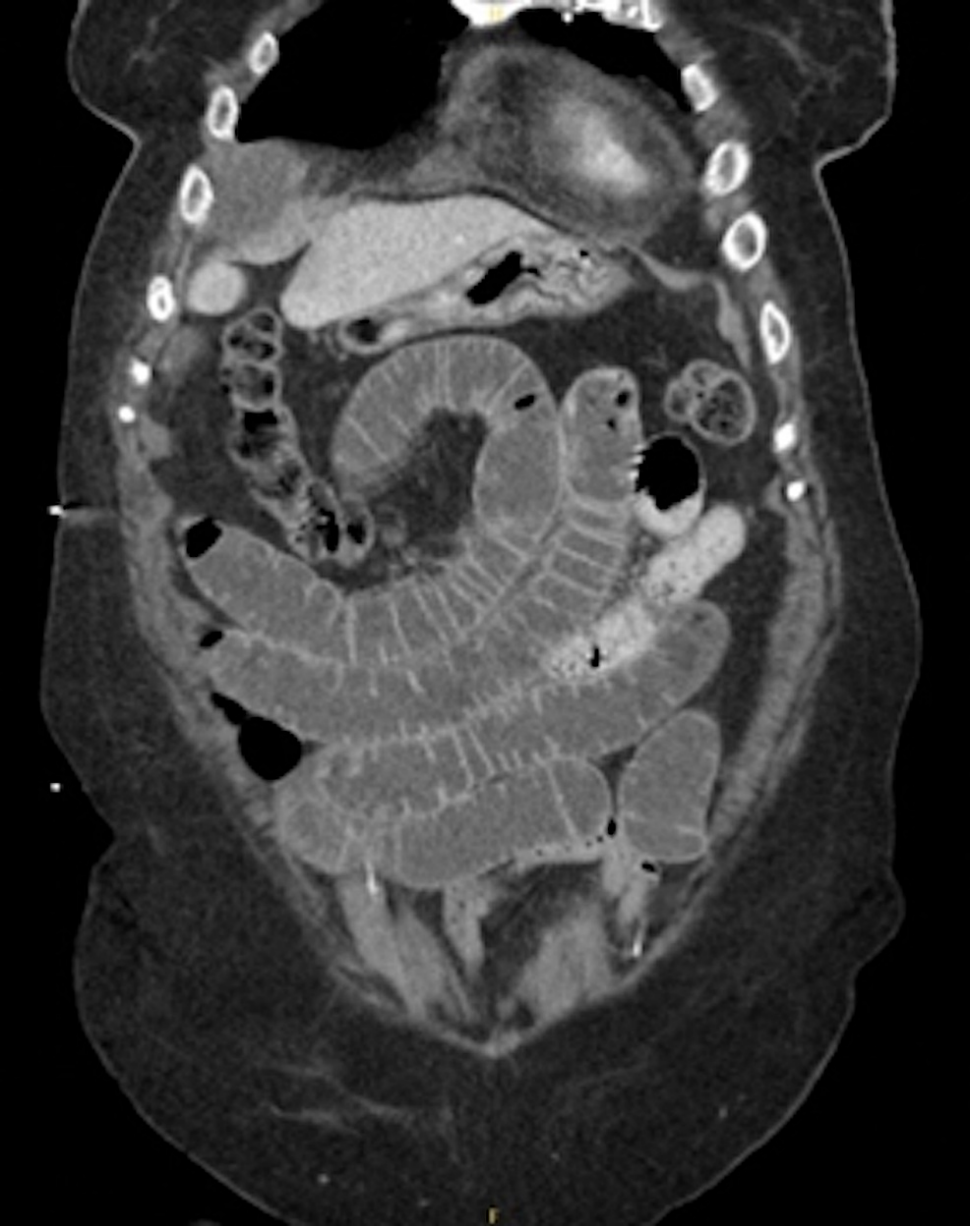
CT abdomen and pelvis showing a small bowel obstruction as evidenced by the inflammatory stranding and tethered loops
